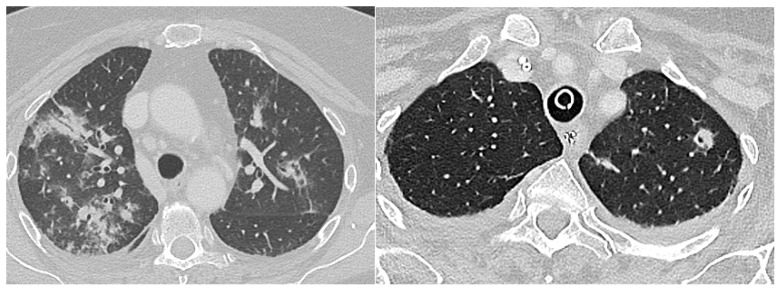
Chest CT. (Left): small patchy consolidations in the upper lobes, more pronounced on the right side, with mild associated ground-glass opacity and air bronchograms, suggestive of Staphylococcus aureus pneumonia. (Right): small consolidation with a tiny cavity in the left upper lobe in another patient with *Staphylococcus aureus* infection. Representative image from the anonymized institutional radiology archive of University Hospital Frankfurt.
